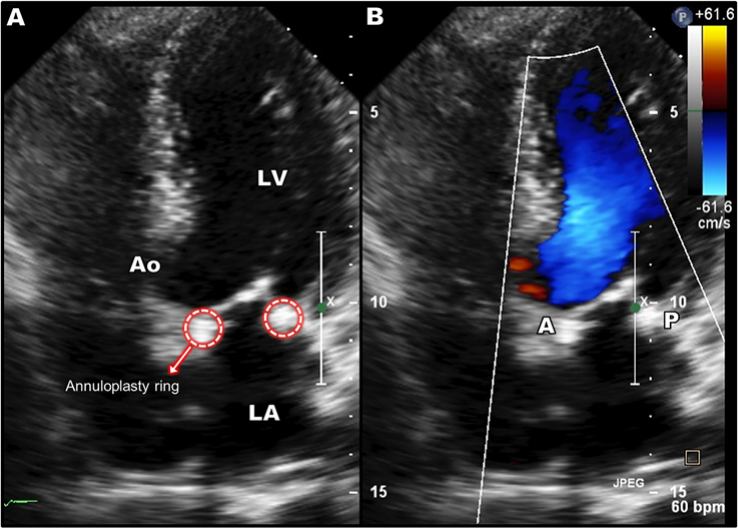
Post-repair Doppler ultrasound data following the actual surgical repair (quadrangular resection and ring annuloplasty) to treat the posterior MV prolapse.
